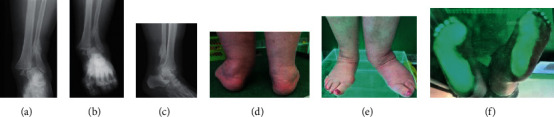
(a– f) Results of imaging tests (X-ray) and podoscopic tests: patient no. 2. (a) Posterior-anterior X-ray; fracture of the medial ankle and fracture of the distal end of the fibula. (b) Anterior-posterior X-ray: fracture of the medial ankle, fracture of the distal end of the fibula, overload changes in the phalangeal joints, and inflammation in the metatarsal joints. (c) Lateral X-ray: microfractures of the heel bone and enthesopathy of the plantar fascia: calcaneal spur. (d) Posterior picture: ankle joint and hindfoot evaluation. (e) Anterior picture of the feet: assessment of toe positioning and deformation. (f) Plantar photo on the podoscope: assessment of plantar part of the foot, height measurement of the longitudinal arch.
